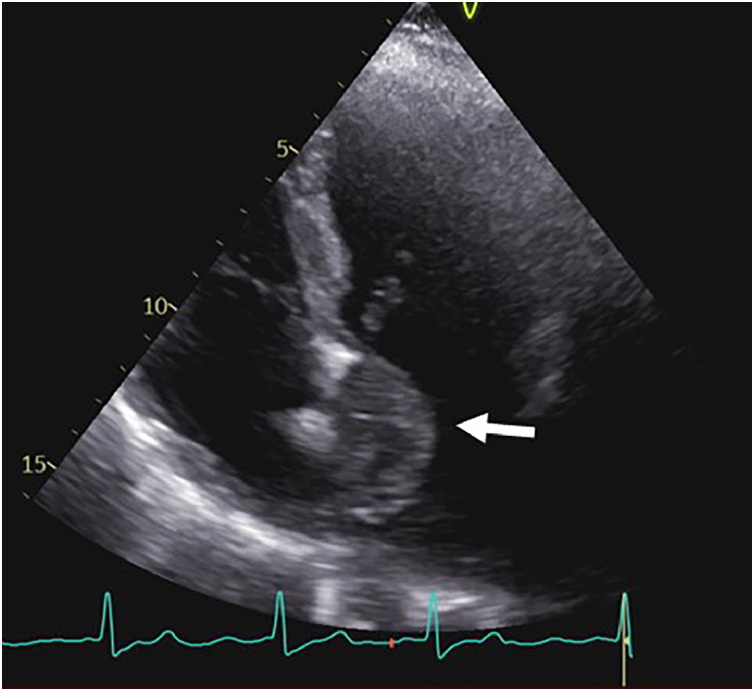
Transthoracic echocardiography (TTE) demonstrating a large, heterogeneous, multilobulated, semimobile mass with attachment to the septal wall of the left atrium and mitral valve annulus in a 4-chamber view (arrow).
